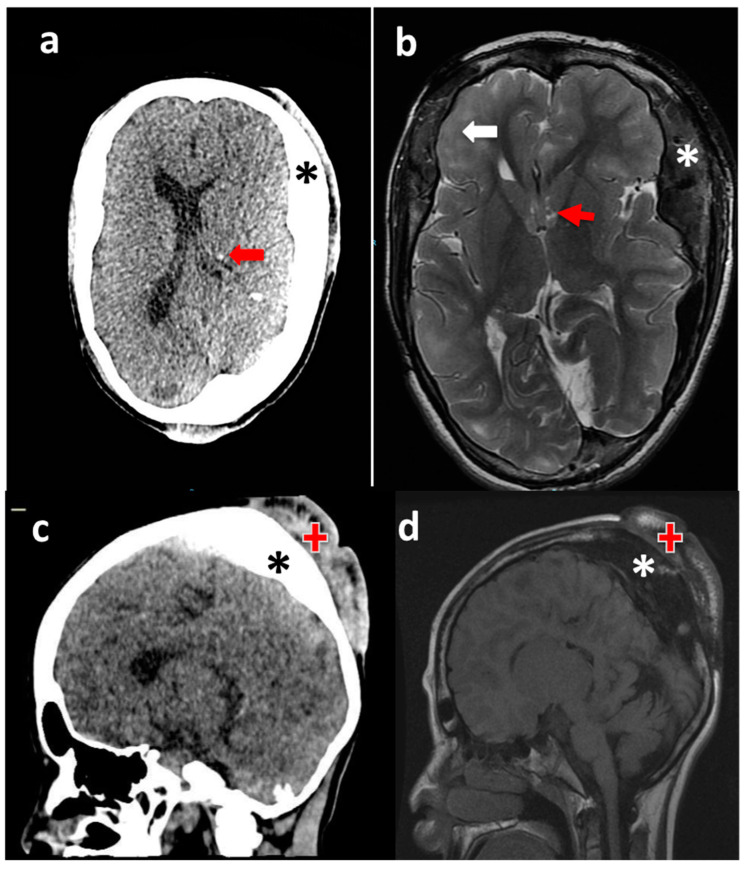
Cerebral images of the patient. (a) CT scan of the skull, showing enlargement of the diploe (asterisk) and calcified subependymal nodules (red arrow). (b) Brain MRI showing an atypical hyperostosis of the diploe (asterisk) with asymmetry of the brain parenchyma, cortical tubers (white arrow) and subependymal nodules (red arrow). (c) Comparison of CT and (d) MRI showing hyperostosis of the diploe (asterisks) and the lack of connection of the FCP (red crosses) with the calvaria and brain.
